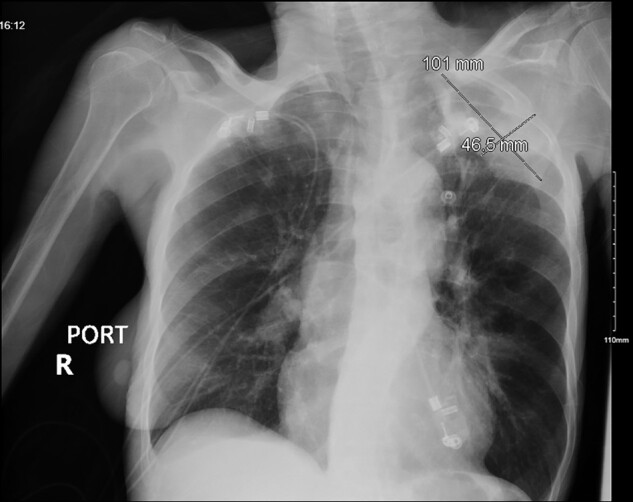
Chest x-ray. 4.6 × 10 cm mass is seen in the left upper lobe.
